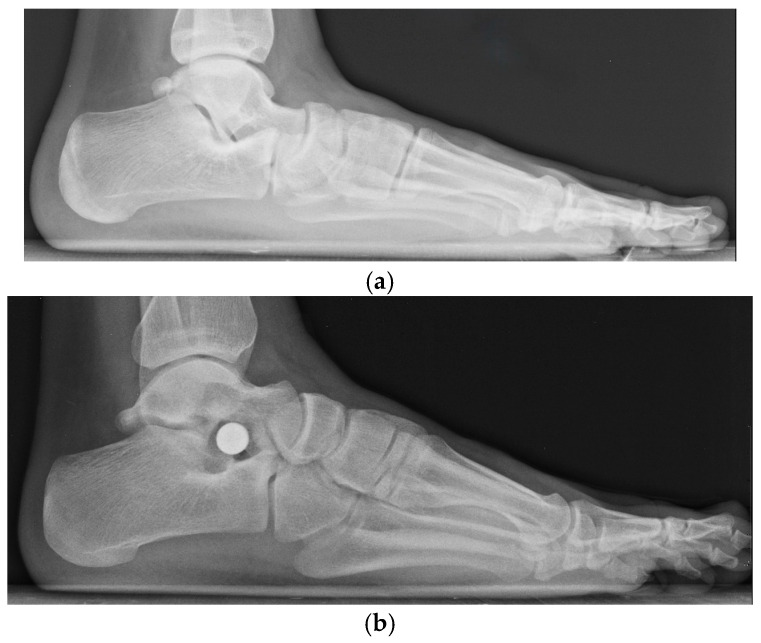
(a) Standing lateral radiograph of the left symptomatic flatfoot of a 12-year-old girl. Meary’s and pitch angles measured, respectively, −16° and 6°. (b) The same foot four months after the application of an endosinotarsal implant. The foot was asymptomatic, and Meary’s and pitch angles measured, respectively, 0° and 15°.
